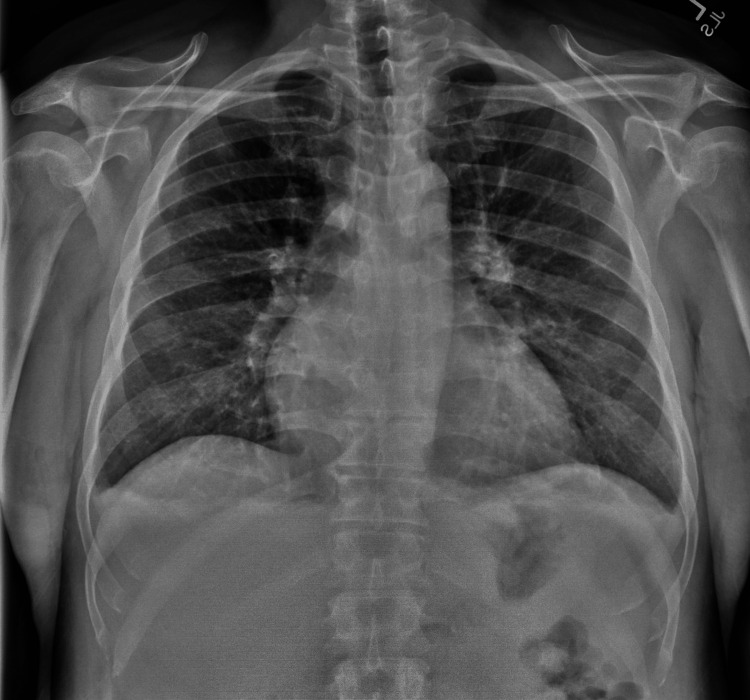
The chest X-ray upon arrival at the emergency department.
